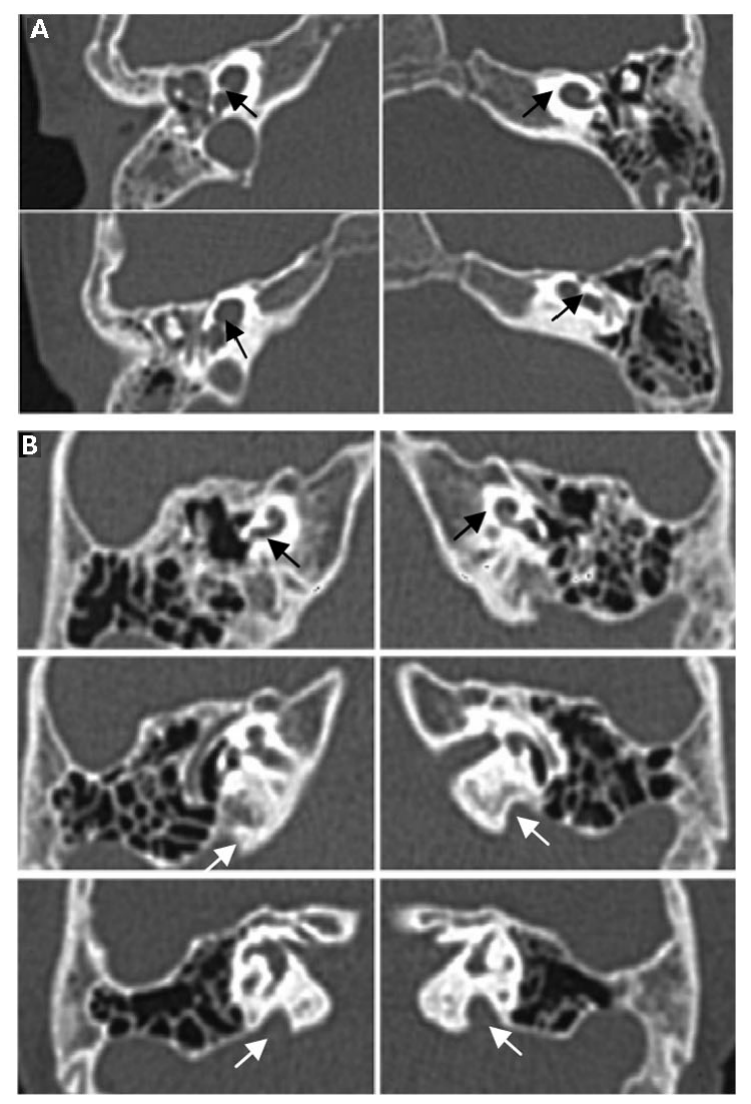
A 1124A>G/1409G>A. (Patient 17). The black arrows in the CT picture showed the common cystic cavity of cochlea and vestibule. B 1472T>C/wt. (Patient 18). The white arrows in the CT picture showed the hypolastic cochlea (Mondini). The black arrows in the CT picture showed EVA.
